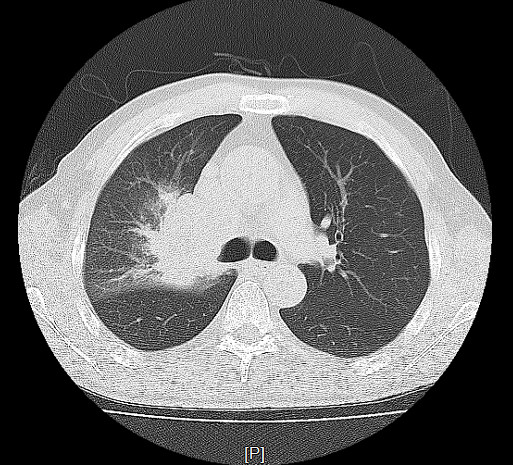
A shadow in the right upper lobe (Lung CT).
